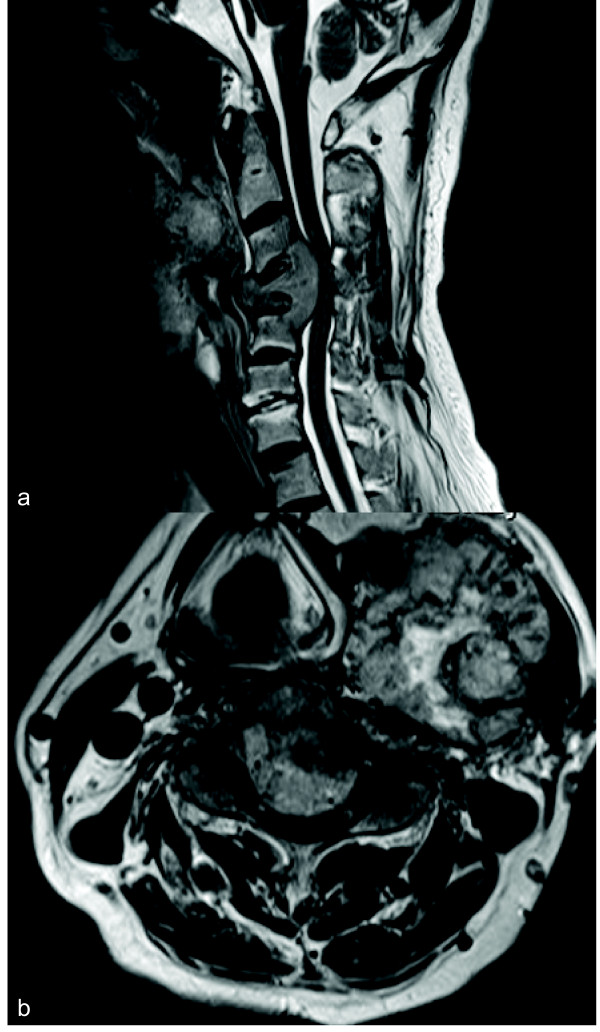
Sagittal (a) and axial (b) T2-weighted magnetic resonance image displaying marked C4 destruction and severe canal stenosis.
